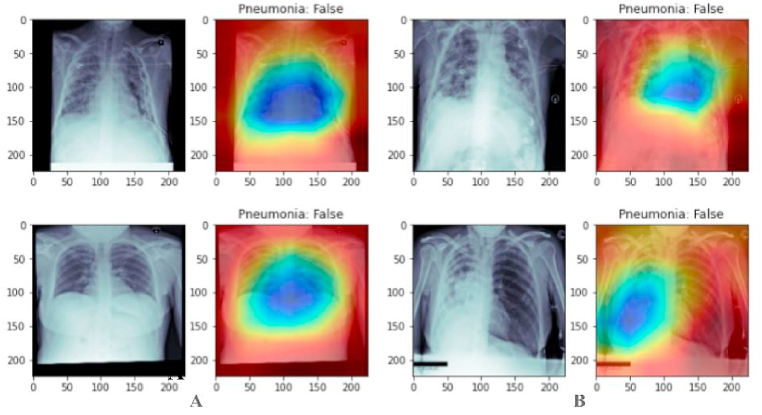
Classification result of chest X-rays for negative pneumonia using our capsule networks cluster model. In Pnaels “A” and “B” the right sides of images are the original image while the left sides of the images shows the unavailability of pneumonia with them as detected by our model.
